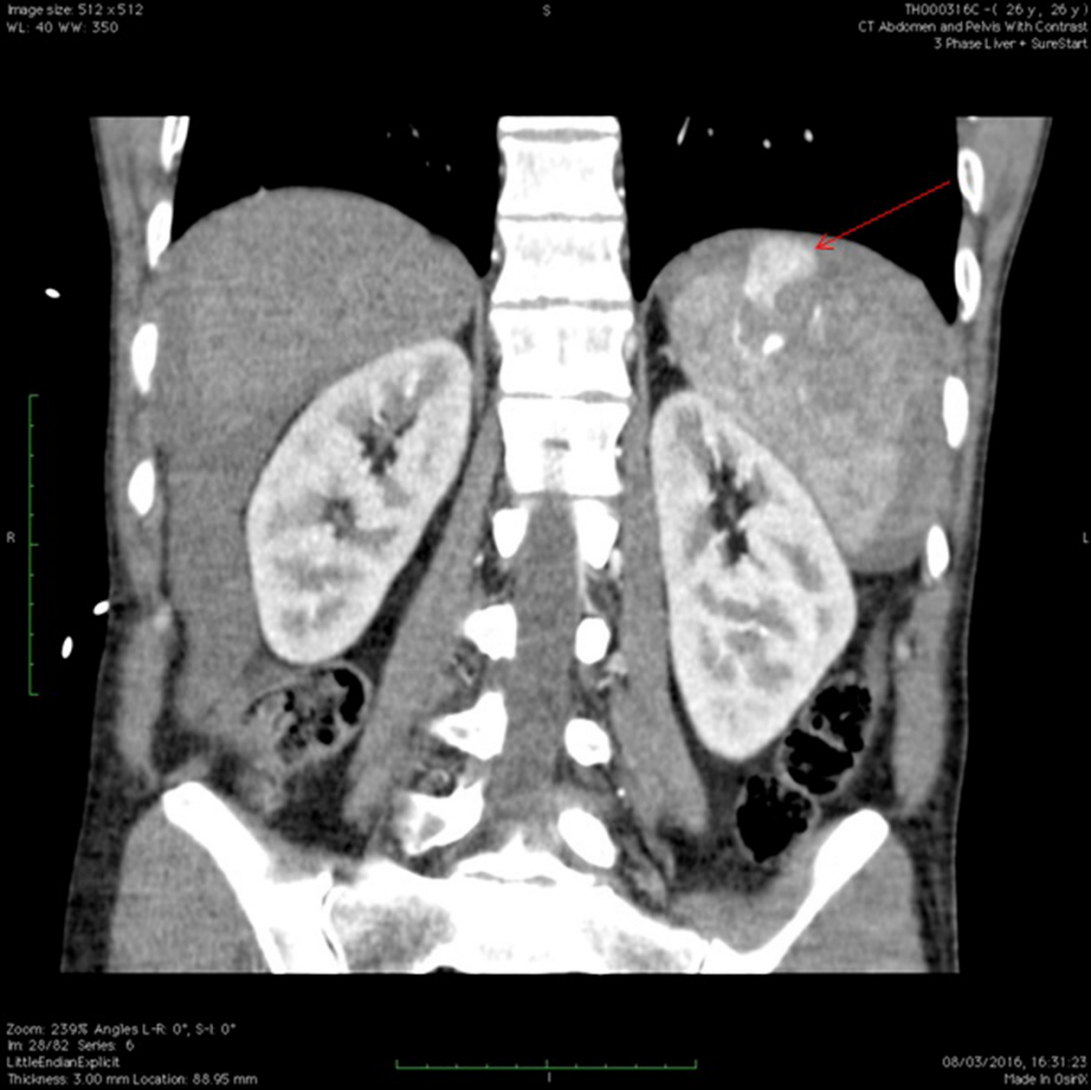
Coronal view of repeat CT scan of abdomen/pelvis with contrast. Active bleeding was still seen at the spleen; however, there was now a new extravasation of contrast (red arrow), indicating splenic capsular rupture.
